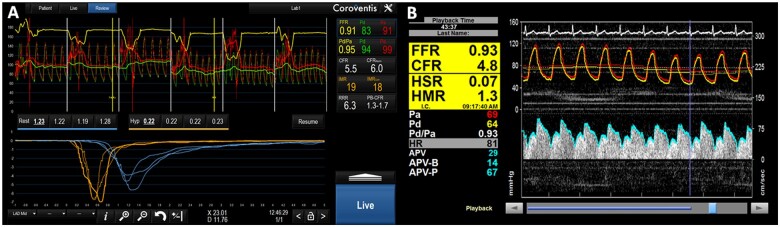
Coronary haemodynamic evaluation with dual sensor coronary wire. (*A*) Coronary pressure and thermodilution. (*B*) Coronary pressure and Doppler.
